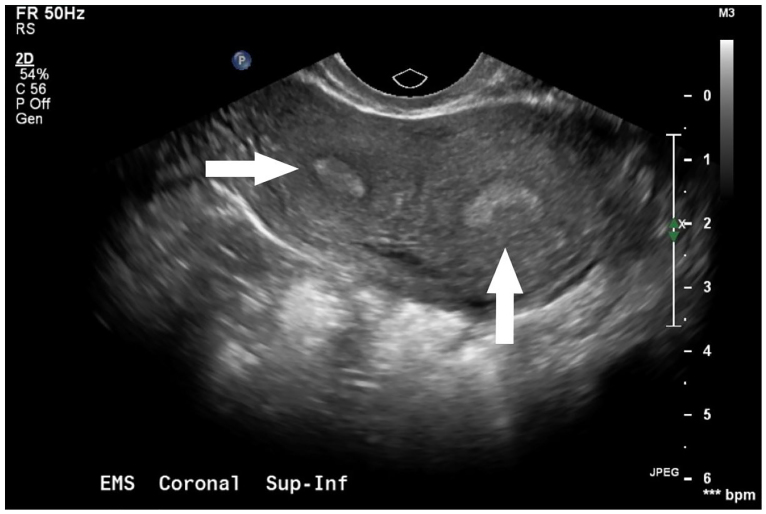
Transvaginal ultrasound demonstrating a gestational sac (arrow) in the left endocervical canal.
